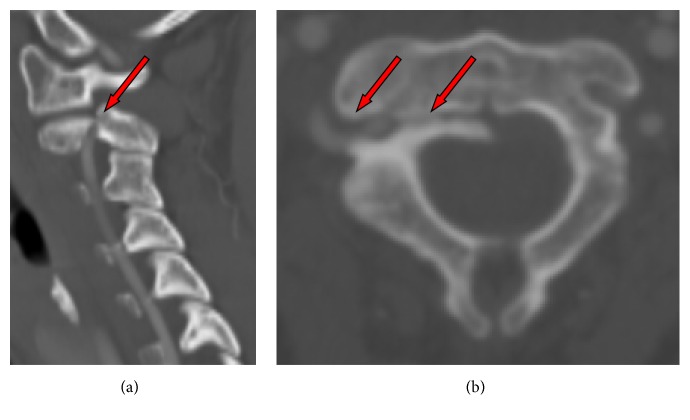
CT with contrast of fracture featuring a partial ossification and artery dislocation after three months: (a) sagittal view and (b) axial view.
